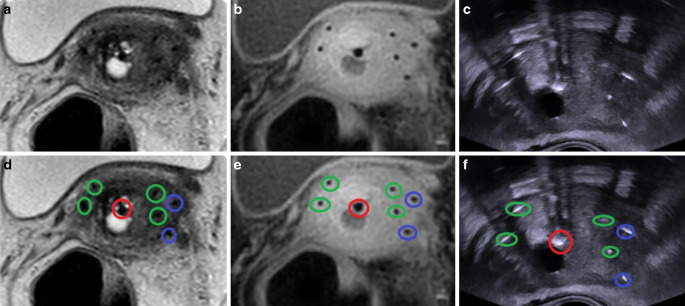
Example of a cervical cancer patient with the applicator as well as four straight and two oblique needles in place. The T2-weighted axial MRI in a and d, T1-weighted axial MRI in the b and e, and the corresponding axial transrectal ultrasound image in c and f. Tandem (*red*), straight needles (*green*), and oblique needles (*blue*) are marked in d–f
